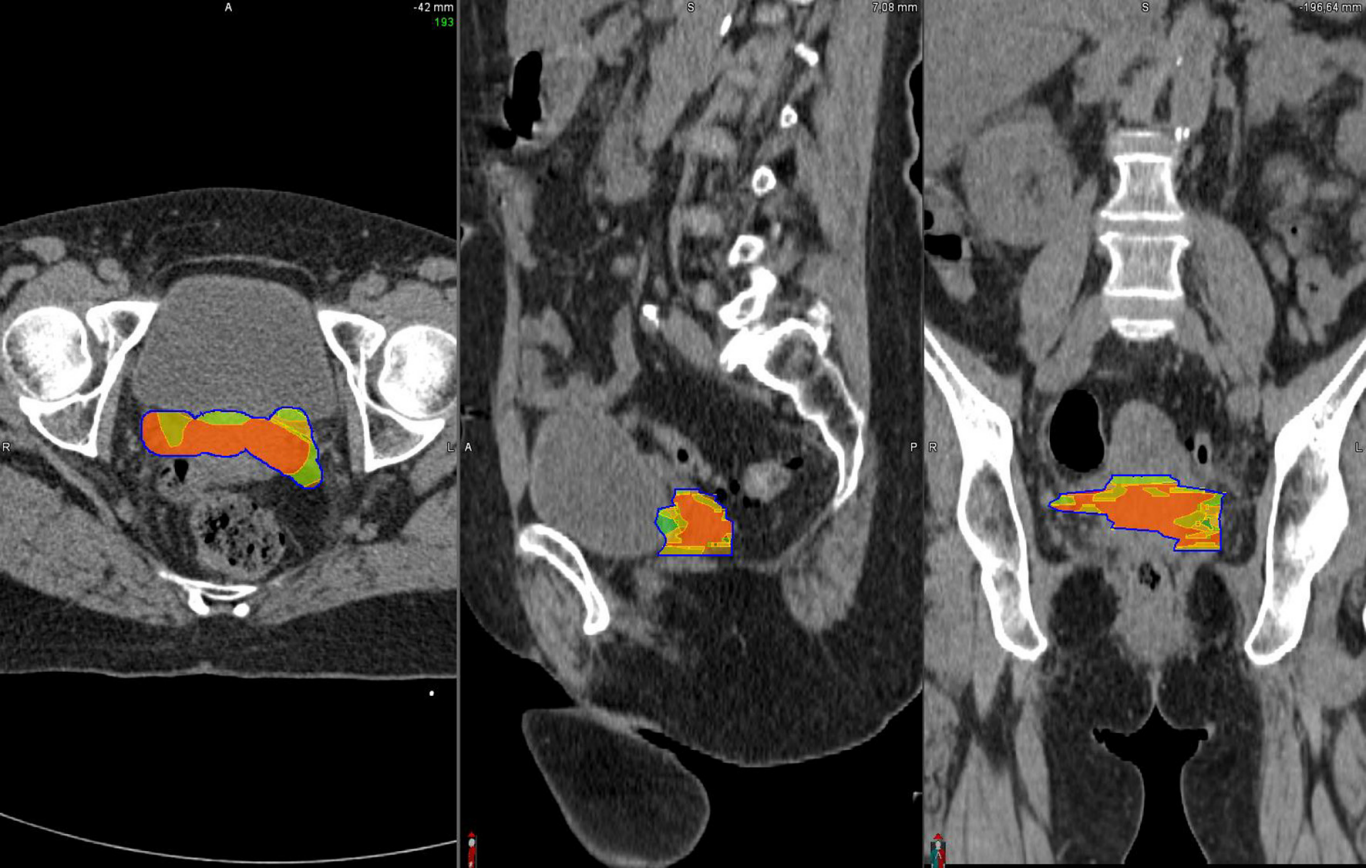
Spatial distribution of all hotspots obtained after deformable registration of the PET imaging sets with a single planning CT. Volumes in red representing the presence of > 75% hotspots, orange 50–75%, yellow 25–50% and green < 25%. (For interpretation of the references to colour in this figure legend, the reader is referred to the web version of this article.)
